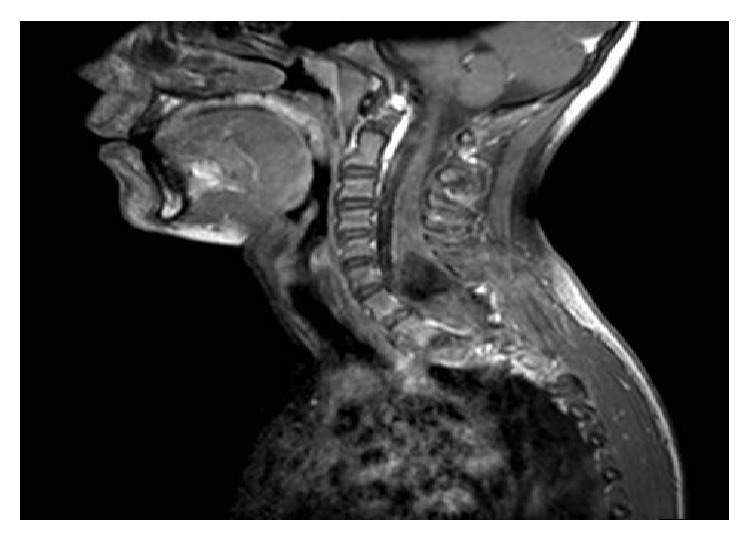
Sagittal fat saturated postcontrast spin-echo (SE) T1-weighted MRI of the cervical spine demonstrates a large expansile mixed solid and cystic intra-axial mass of the spinal cord extending from C5 to T1 level. Both the upper cystic and the lower solid components do not enhance.
